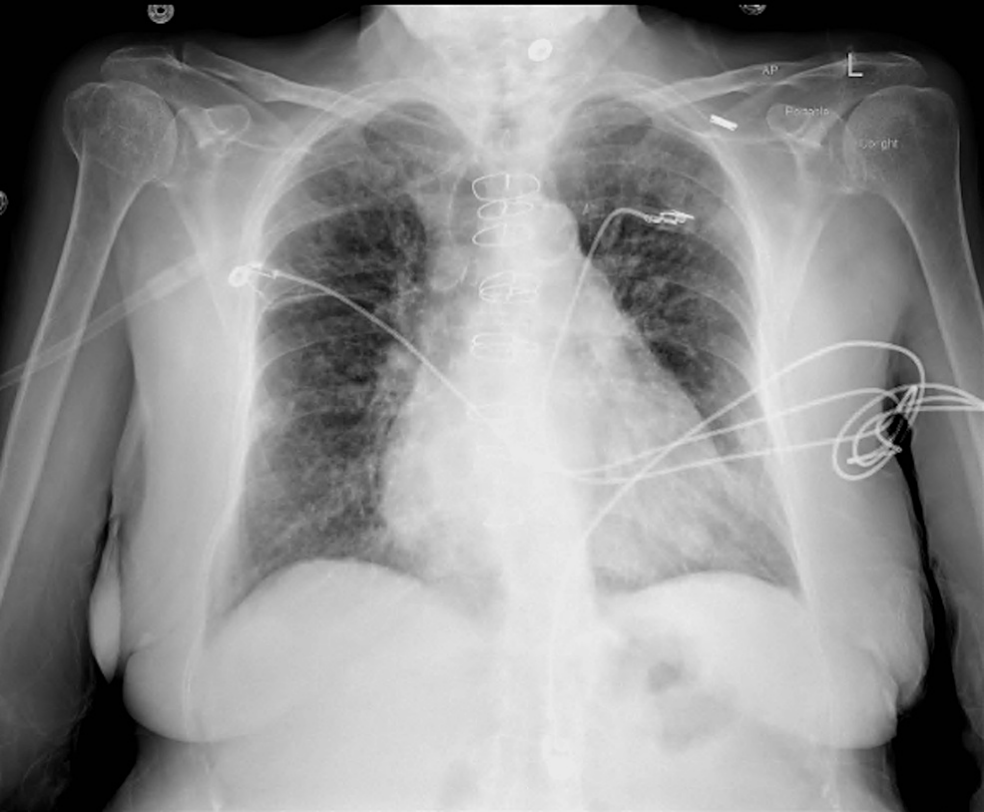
Chest X-ray on the third admission demonstrated the progression of reticulonodular infiltrates bilaterally.
